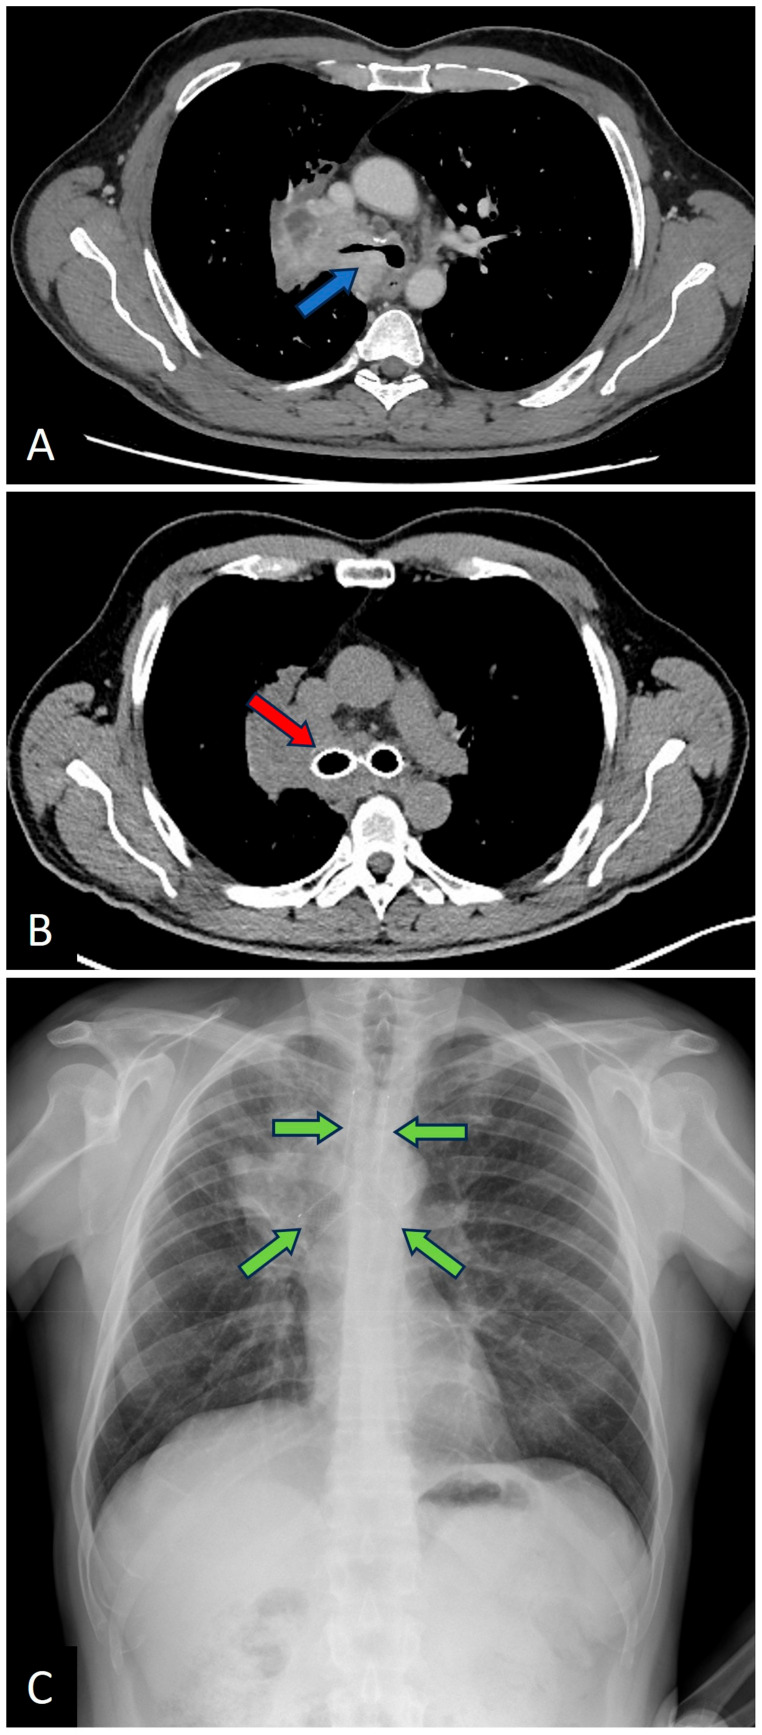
(A) Chest CT showing obstruction of the right main bronchus and carina (blue arrow); (B) Follow-up chest CT after Y-stent placement (red arrow); (C) Chest X-ray showing the same case (stent clearly visible, green arrows).
